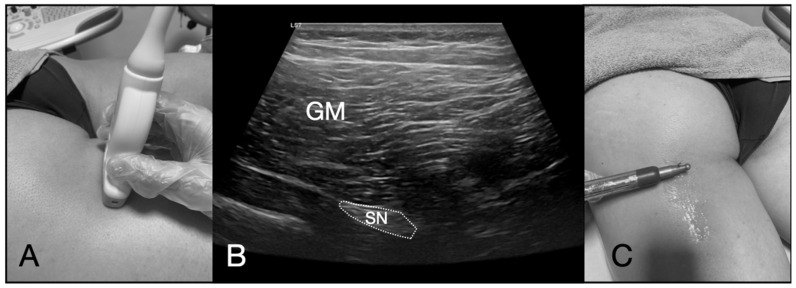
Ultrasound-guided percutaneous neuromodulation. (A) Proximal location on the sciatic nerve; (B) ultrasound image of the sciatic nerve; (C) electrical stimulation to the sciatic nerve using a needle. Abbreviations: GM, gluteus maximus; SN, the sciatic nerve.
